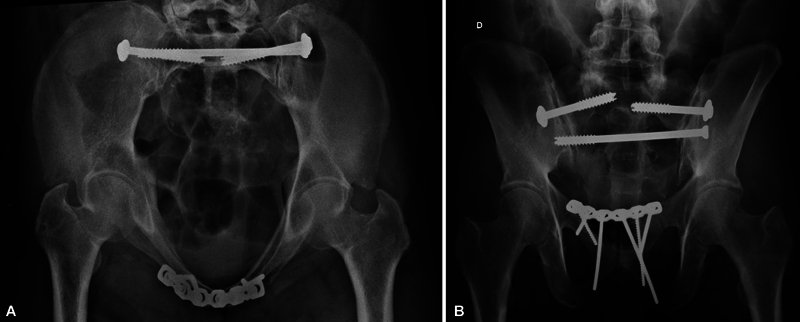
Case example (postoperative radiographs). Postoperative images in inlet (A) and outlet (B) views demonstrating the iliosacral fixation in S1 bilaterally and transiliac-transsacral fixation in S2.
